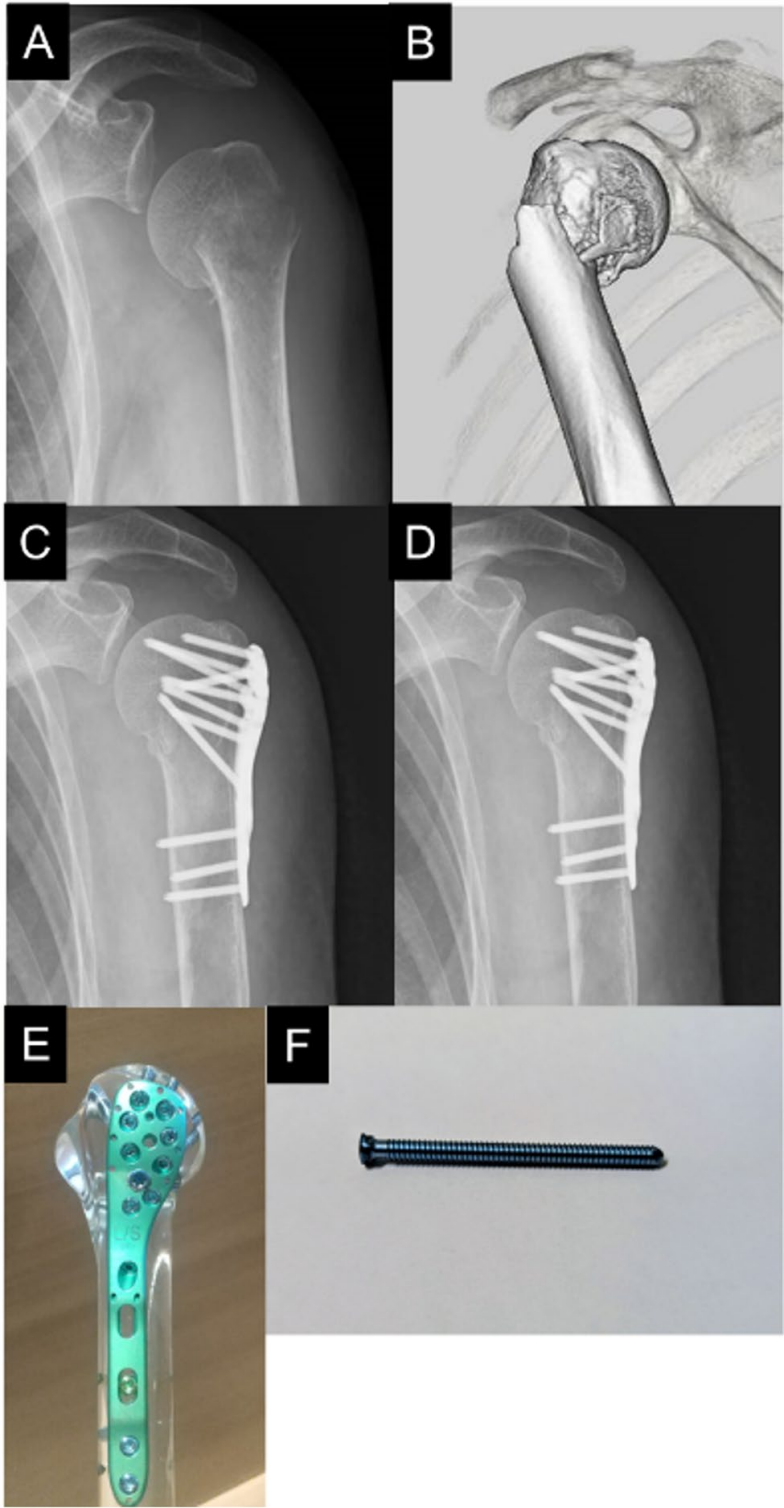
Clinical images and photographs of the plate fixation using MODE^®^ plate with all screws. Preoperative radiograph (A), three-dimentional compuded tomography image (B), and radiograph immediately after surgery (C) and 1 year at postoperatively (D) of the patient who underwent plate fixation using MODE^®^ plate with all screws. Photograph of MODE^®^ plate (E) and proximal screw (F)
